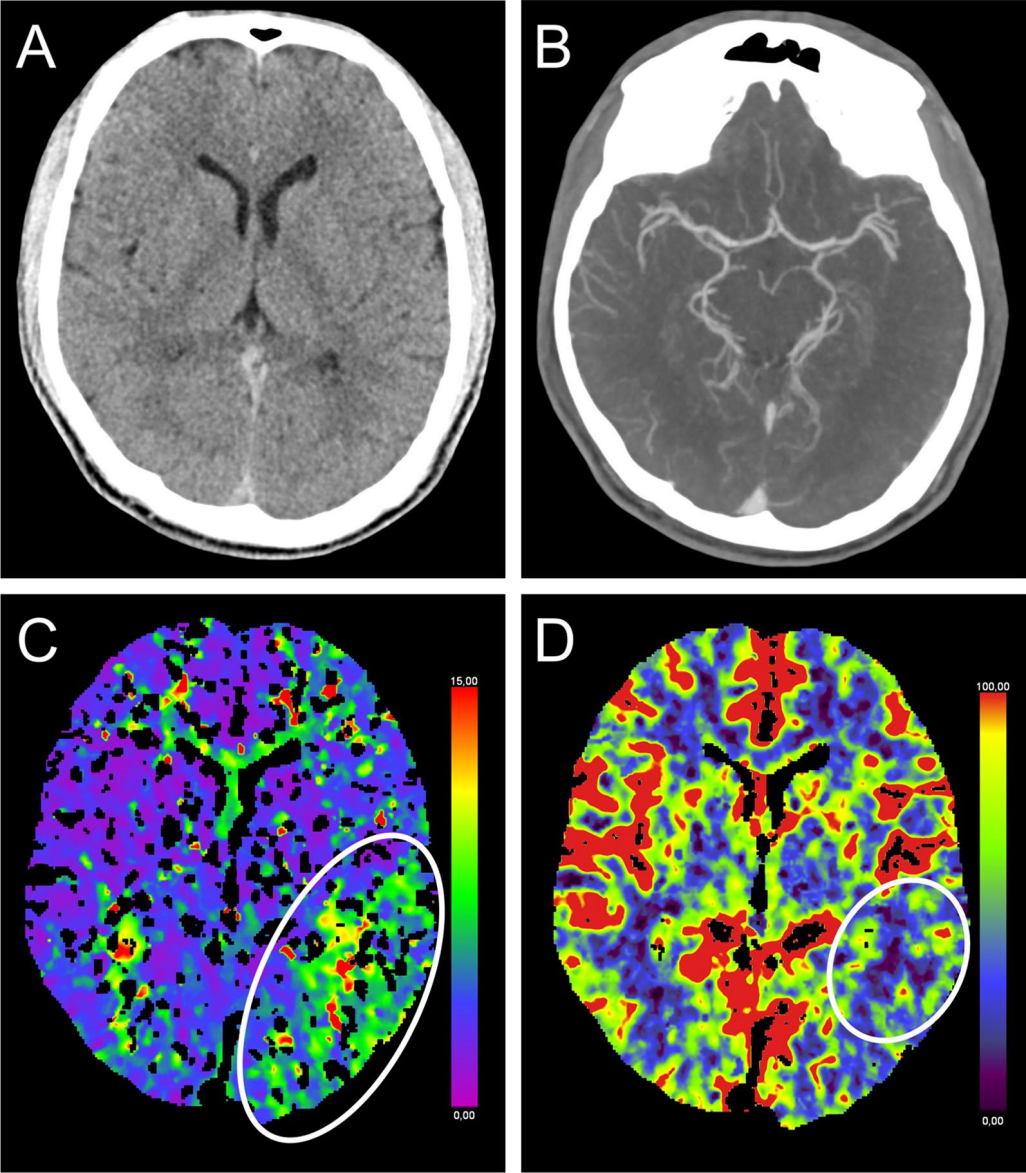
NcCT, CTA, and CTP of patient 1. Exemplary cuts of first ncCT (A), CTA (B), and CTP (C–D) 87 min after onset of aphasia. CTA without relevant stenosis of extra- or intracranial vessels (B). Follow-up ncCT 31 h after onset (not shown) did not show infarct demarcation compared to first ncCT (A). Hypoperfusion parieto-temporal on TTD (C) and smaller reduced perfusion on CBF (D) marked with circle. Only mild delay (2 s) was detected in corresponding TTP. CBF, cerebral blood flow; CT, computed tomography; CTA, CT angiography; CTP, CT perfusion; ncCT, non-contrast CT; TTD, time-to-drain; and TTP, time-to-peak
